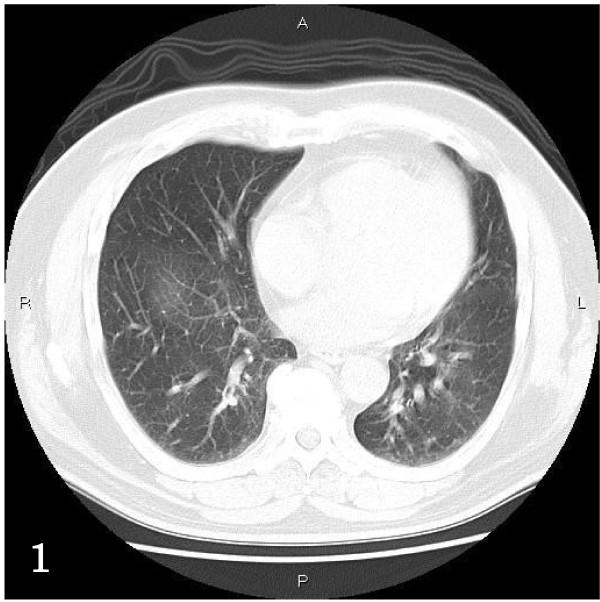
Chest CT. Diffuse reticular opacities are seen in both lungs.
